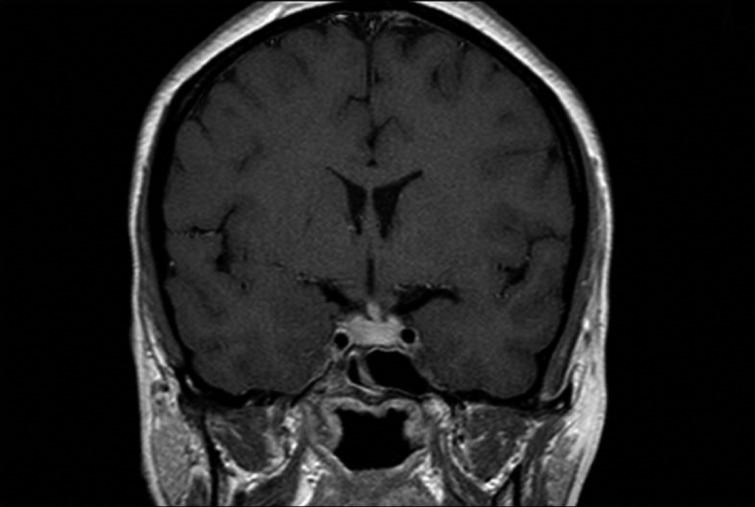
MRI scan of pituitary fossa 7 months after completing 1 year ATT.
